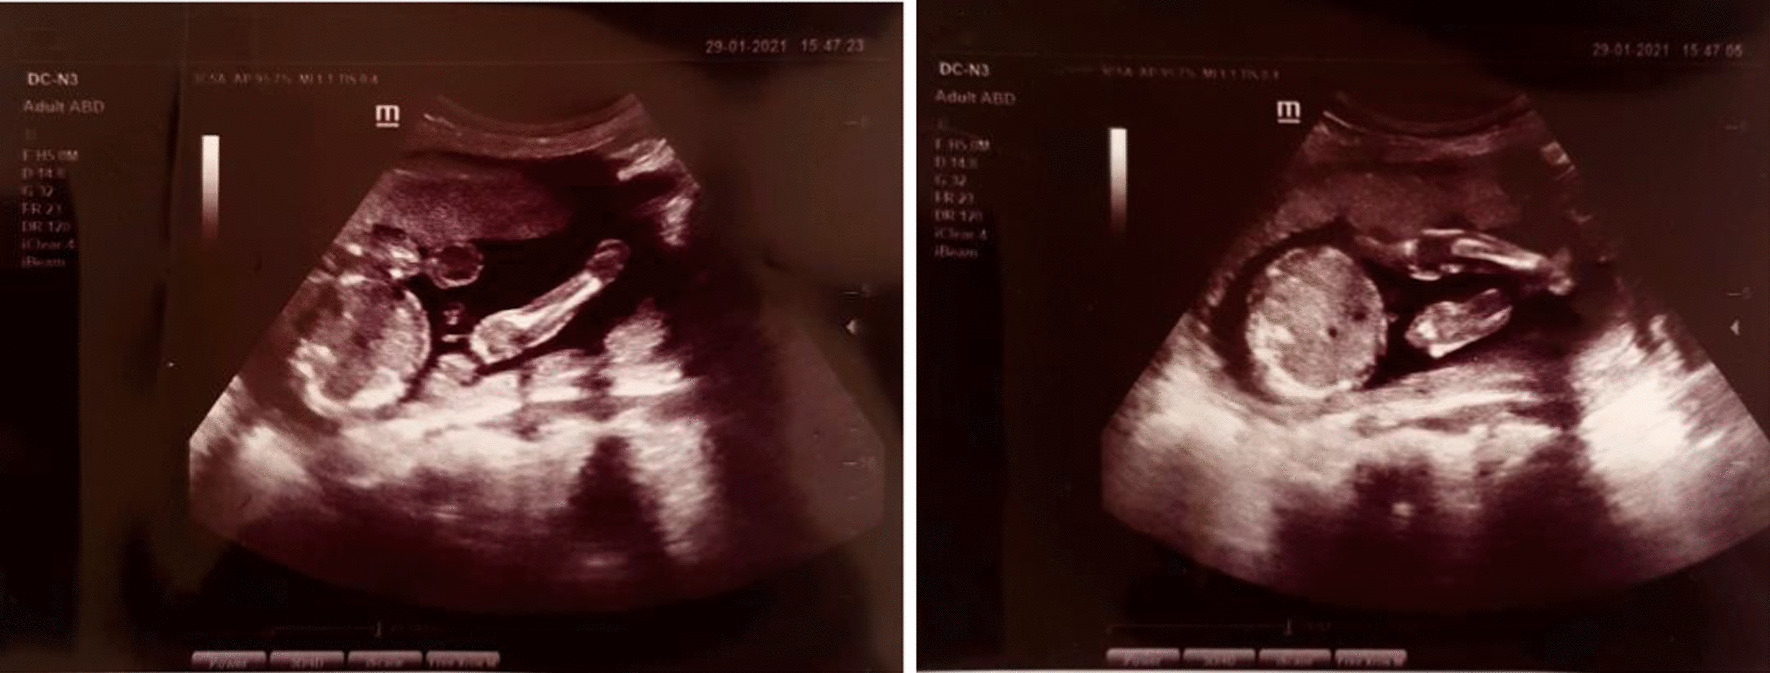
Ultrasound images of second-trimester intrauterine pregnancy at 18 weeks 3 days, which was later terminated
